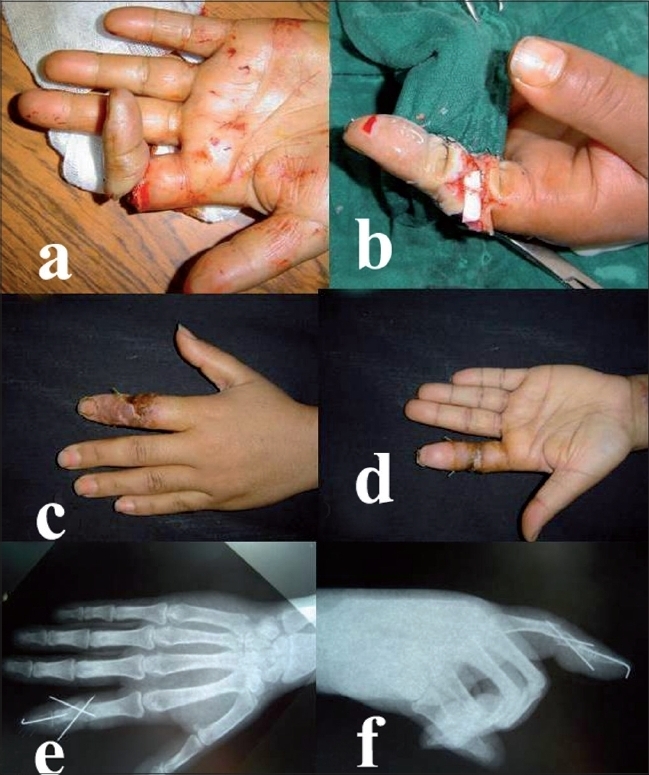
(a) Urbaniak Class III avulsion through proximal interphalangeal joint of left index finger with finger ischemia. Termino terminal anastomosis on the radial collateral artery after shortening arthrodesis of the proximal interphalangeal joint. Two weeks postoperative (c-d) aspect of the left hand after the procedure. Postoperative AP (e) and lateral (f) X-rays of left middle ray
